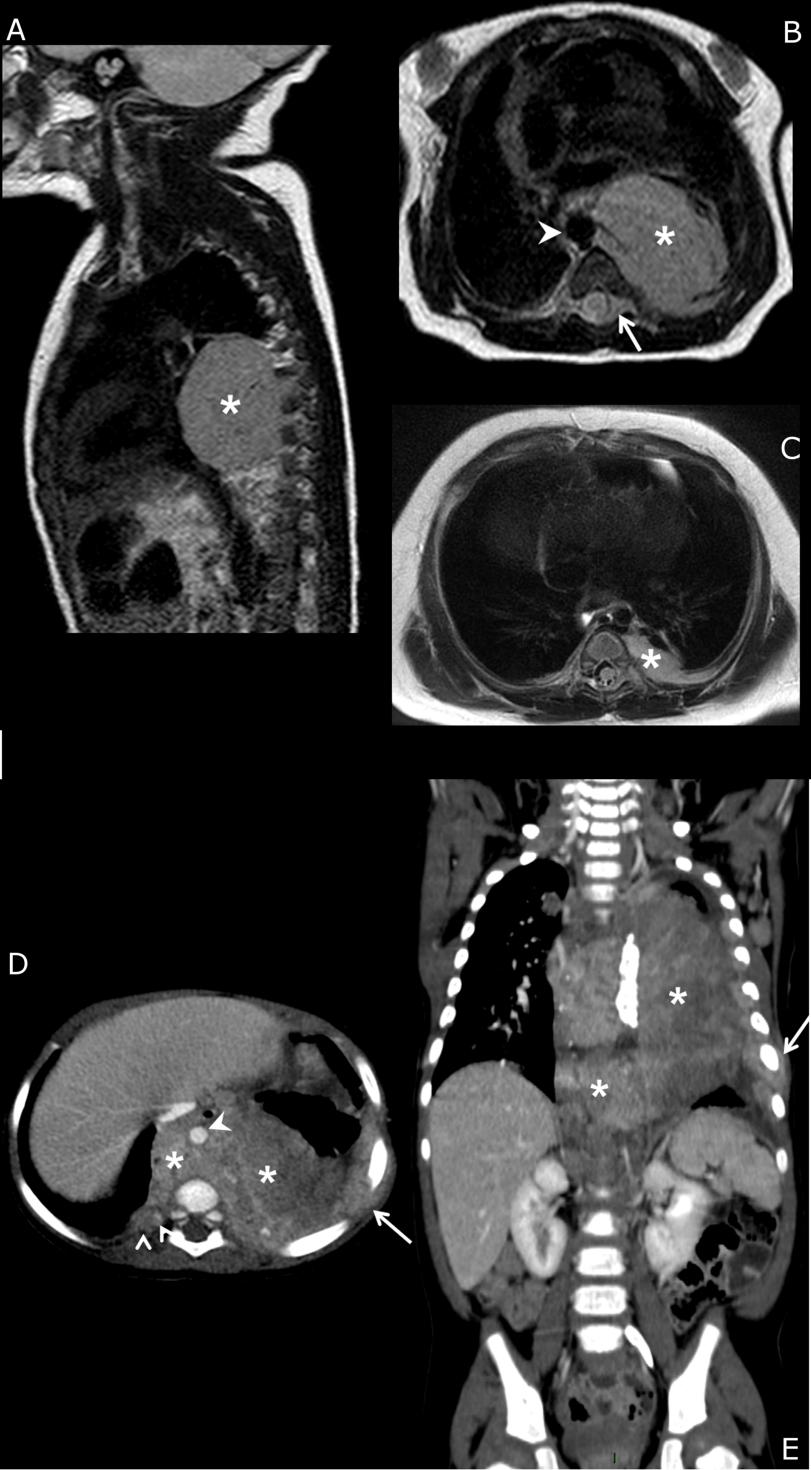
Imaging phenotypes of chest neuroblastomas. (a, b, c) Newborn with L2-stage left posterior mediastinal NCA neuroblastoma. MRI at diagnosis (a, b: sagittal and axial T2-weighted sequences). The primary tumor is a unique well-delineated mass (*) with focal contact with the thoracic aorta (arrowhead) and intra-spinal extension (arrow). Follow-up MRI 3 months later (c) after neoadjuvant chemotherapy (2 courses of cyclophosphamide-vincristine and 2 courses of etoposide-carboplatin) shows the tumor residue precisely located at the costo-vertebral junction, i.e., a paravertebral sympathetic chain location. (d, e) 9-year-old girl with M-stage mediastinal SCA neuroblastoma. Enhanced CT scan at diagnosis (axial and coronal views). The primary tumor (*) is ill-defined and diffusely infiltrates the posterior mediastinum, pleura and chest wall (arrows), crosses the midline and encases the thoracic aorta (arrowhead). The presumed origins are the mediastinal sympathetic fibers surrounding the descending aorta.
